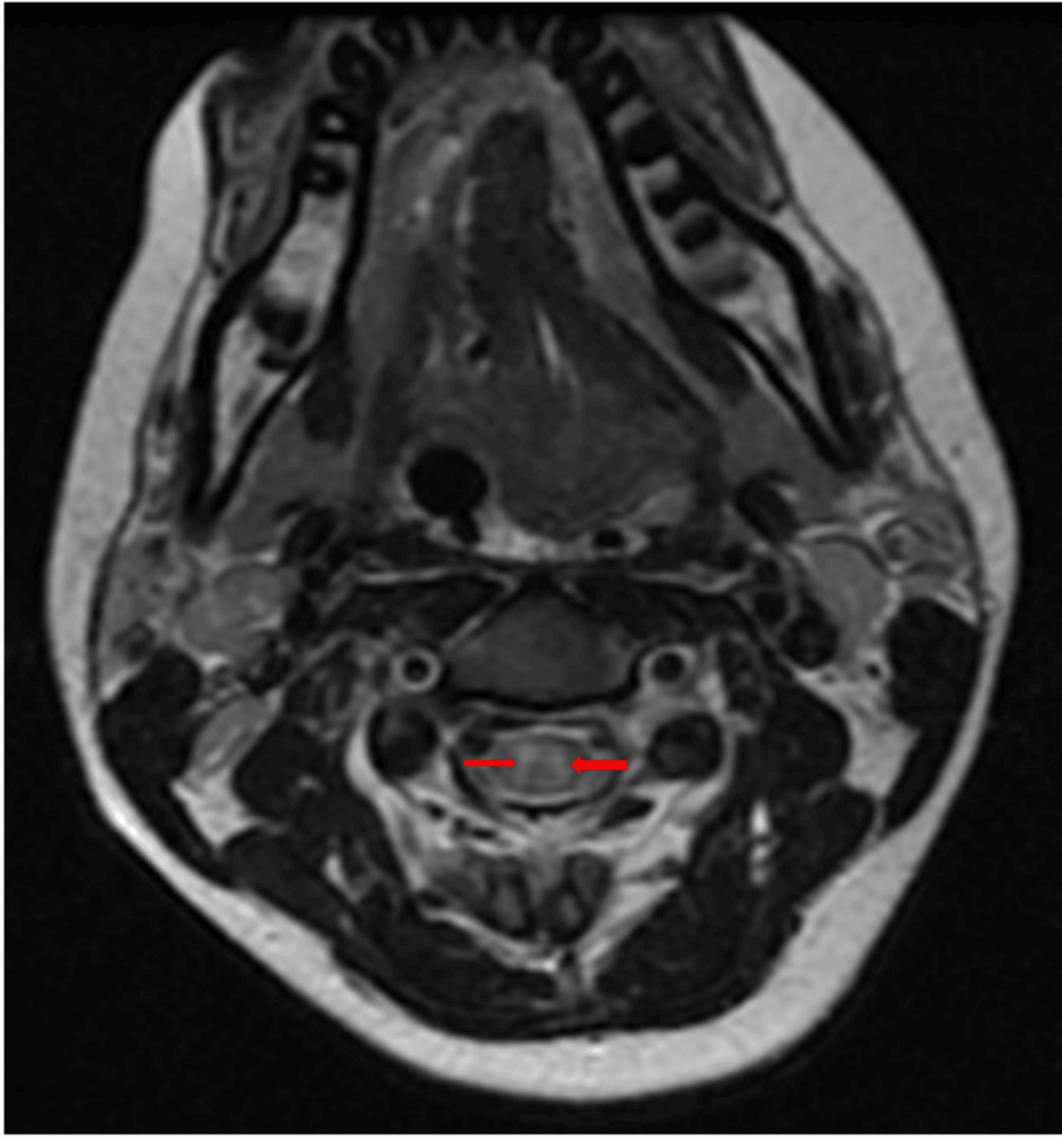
Axial T2-weighted MRI at C5 excluding compressive pathology Axial T2-weighted image at C5 showing diffuse central intramedullary hyperintensity without evidence of extrinsic compression (arrow). MRI: Magnetic resonance imaging
